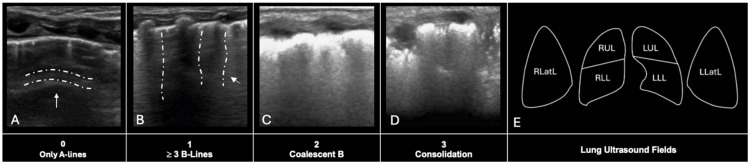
Lung ultrasound scoring at the Montreal Children’s Hospital NICU. (A) A score of 0, characterized by a clear pleural line and the presence of horizontal A-lines (indicated by the arrow) depicting a well-aerated lung; (B) A score of 1, showing at least three well-spaced vertical B-lines (indicated by the arrow); (C) A score of 2, representing confluent/coalescent B-lines; (D) A score of 3, indicating extensive lung consolidation; (E) 6-zones scanned in our protocol, the three sections scanned per lung (typically the upper anterior, lower anterior, and lateral zones) to calculate the total lung ultrasound score.
